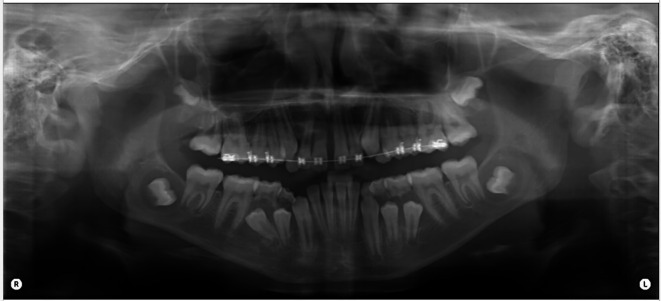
Preoperative panoramic radiograph (OPG).
